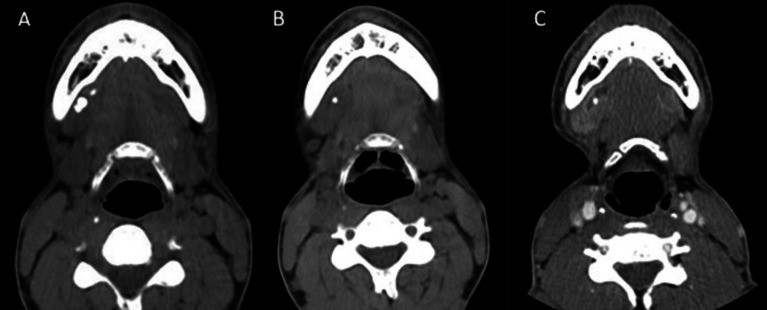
A) Initial axial cervical non-enhanced CT scan with right main and accessory submaxillary glands and lithiasis in the Wharton’s duct. B) Axial cervical non-enhanced CT scan with accessory submaxillary gland and reparative scar tissue after the first intervention. C) Axial cervical CT scan on administration of iv contrast after the second intervention, with absence of the right submaxillary glands, dilation of the right Wharton’s duct and calculus in its interior.
